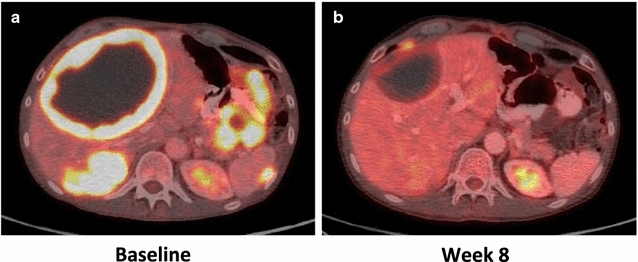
Radiological response of a GIST possessing an ETV6–NTRK3 fusion following treatment with LOXO-101, a selective TRK inhibitor. A 55-year old male with a T3N0M1 small intestine GIST had progression of disease on five lines of tyrosine kinase inhibitors targeting KIT prior to identification of an ETV6–NTRK3 fusion in the tumor. He was enrolled on a Phase I clinical trial of oral LOXO-101 (Loxo Oncology, Stamford, CT), a selective TRK inhibitor. As compared to baseline PET/CT images (a), the tumors had decreased size and FDG-uptake at week 8 (b). At 4 months, the patient had ongoing partial response (44%) according to RECIST 1.1 criteria
